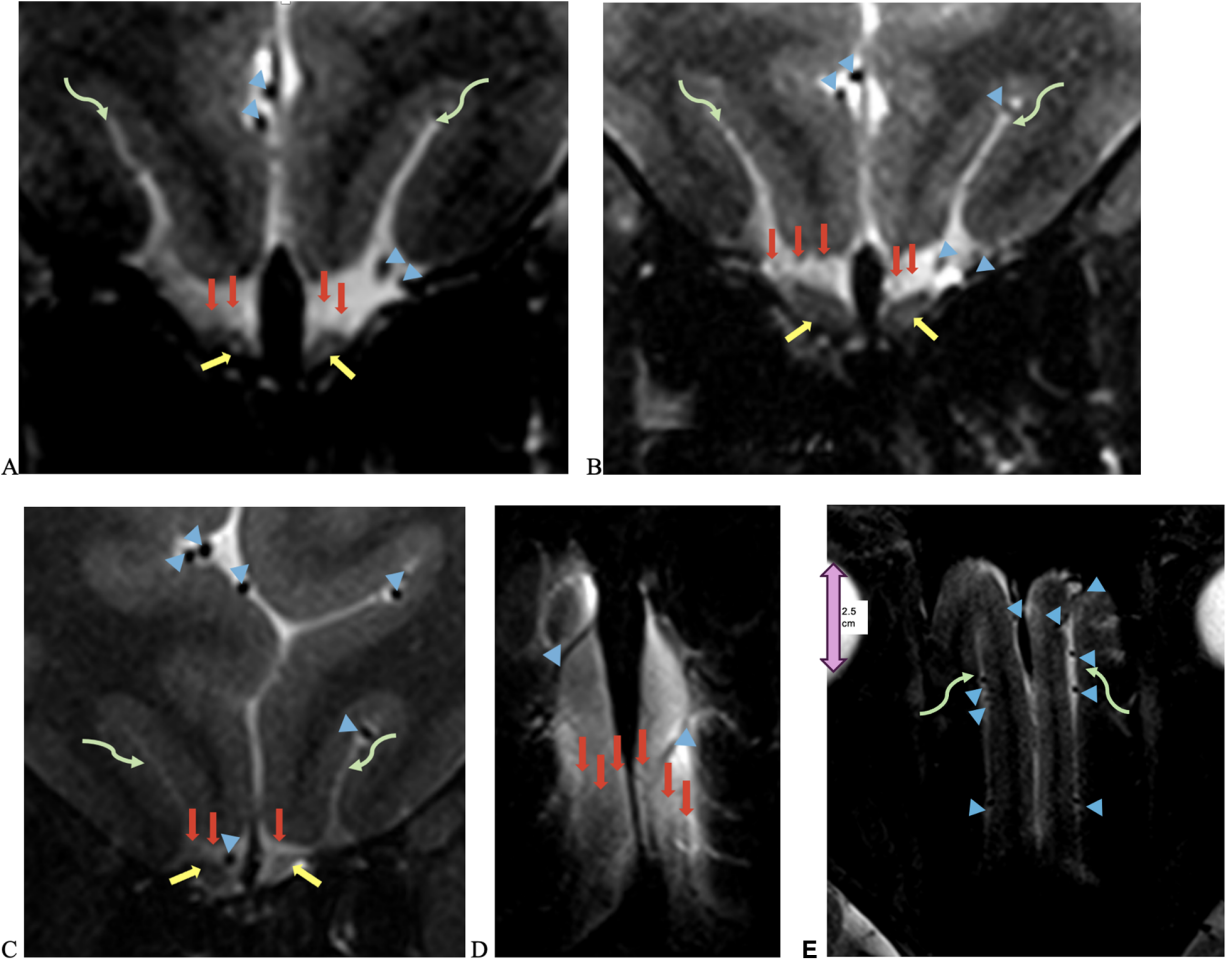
UHF 7-T MRI T2-weighted (A–C) coronal and (D,E) axial scans of a 43-year-old female with anosmia and hypogeusia during and persisting after recovery from COVID-19. Yellow arrows point to olfactory bulbs, red arrows point to the NT, blue arrowheads point to vessels with flow voids, and curved green arrows point to the olfactory sulcus. There is an increased T2 signal in the bilateral olfactory bulbs, with the left greater than right olfactory tracts and NT, as well as left volume loss greater than right volume loss in the left olfactory bulb and tract, with an asymmetric widening of the left olfactory sulcus.
